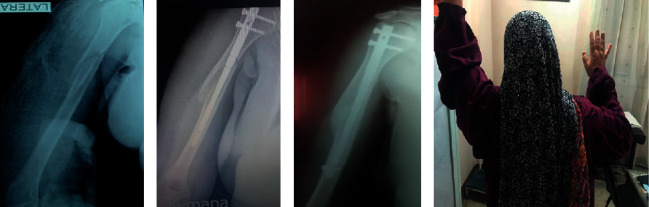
A 48-year-old female had right humeral shaft fracture AO/OTA type 12-A_2_ as shown in the preoperative X-ray (a). She was treated by interlocking nail (b). 8 months after surgery, there was complete bone union (c), but there was limited range of shoulder motion due to impingement and secondary frozen shoulder (d).
